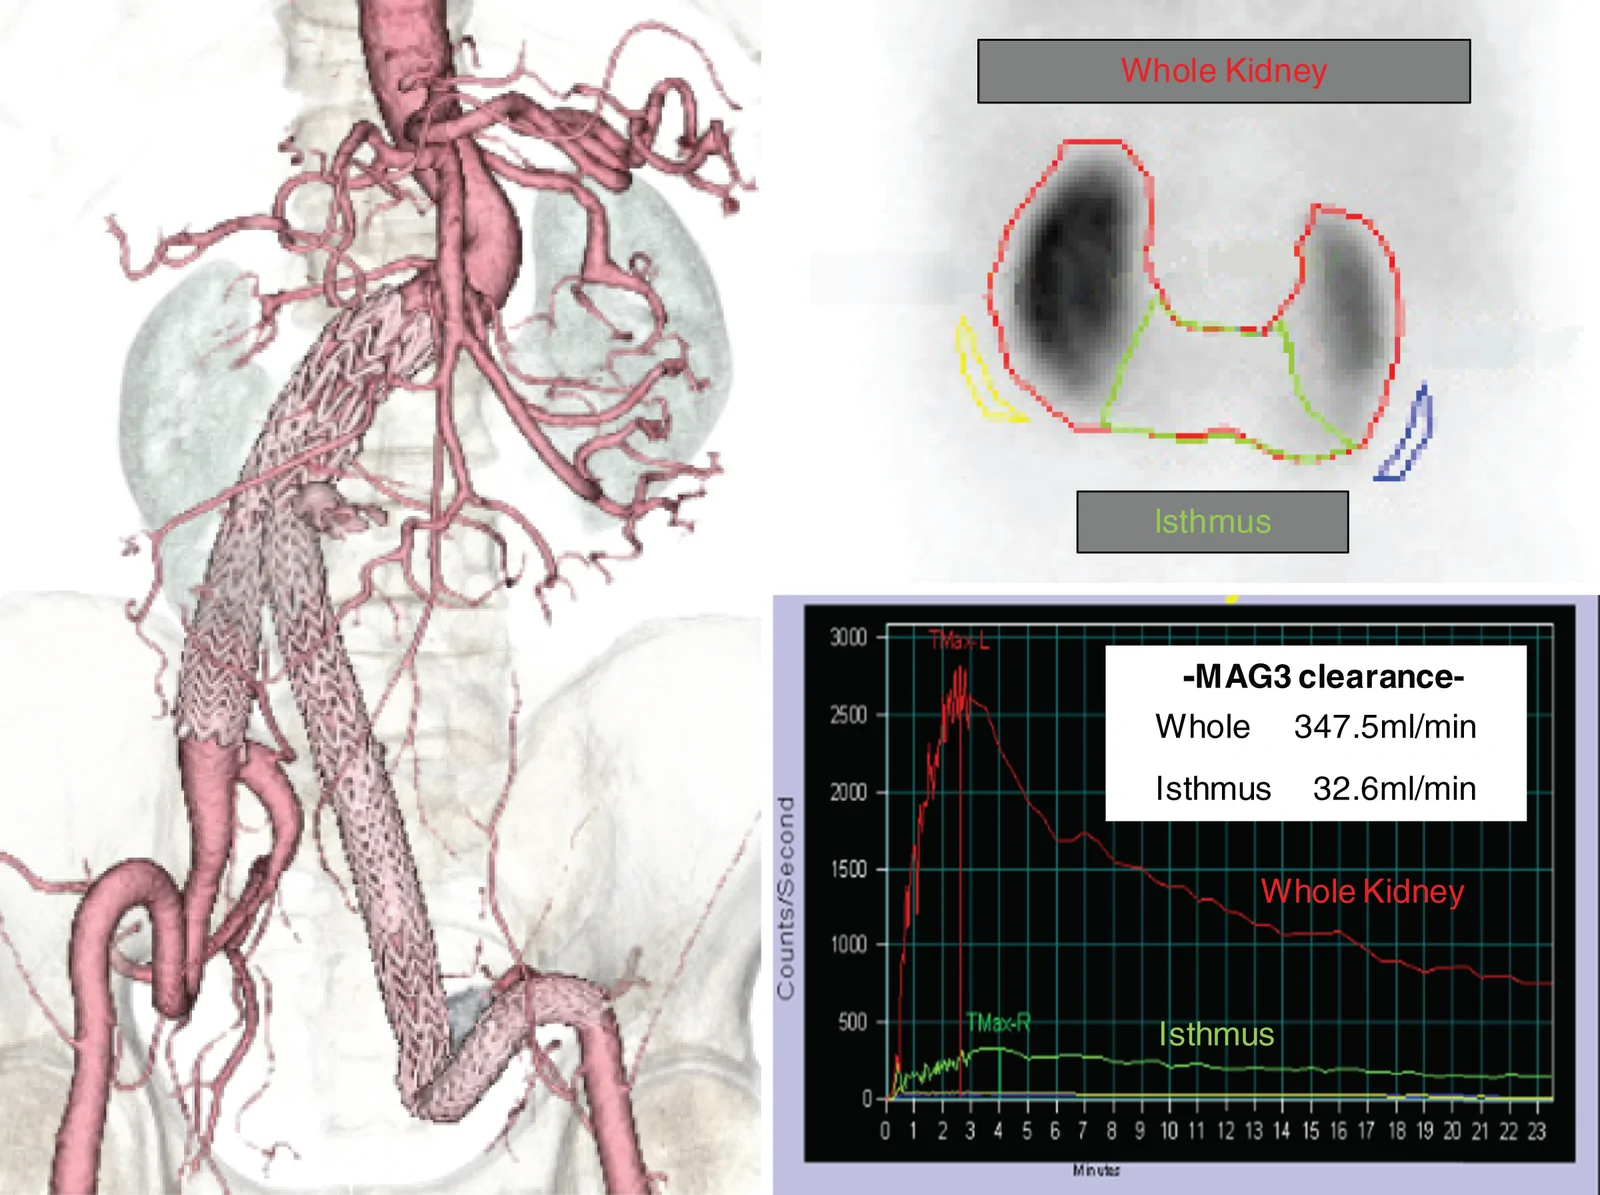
The stent graft was placed in the planned position, and no subsequent endoleak was observed. Renal scintigraphy showed that the post-EVAR MAG3 clearance of the isthmus was 32.6 mL/min, while the sum of the left and right kidneys excluding the isthmus was 314.9 mL/min. EVAR, endovascular aortic repair; MAG3, mercaptoacetyltriglycine
